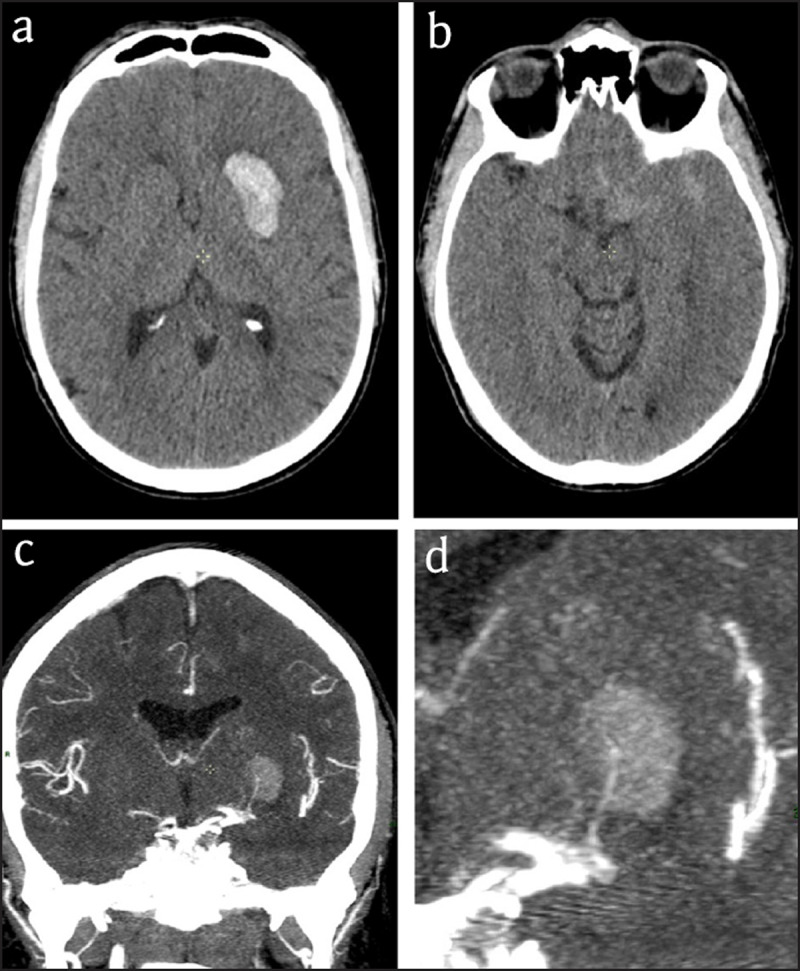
CT. (a) Plain CT: Hemorrhage in the left basal ganglia (Arrow) (b) Plain CT at a lower level: Notice subarachnoid hemorrhage in the cistern of the middle cerebral artery and the insula (arrowheads) (c) CTA: Notice fusiform aneurysm (arrow) on a distal lenticulostriate artery. (d) Magnified view of CTA showing the aneurysm in continuity ith the lenticulostriate artery (arrow).
